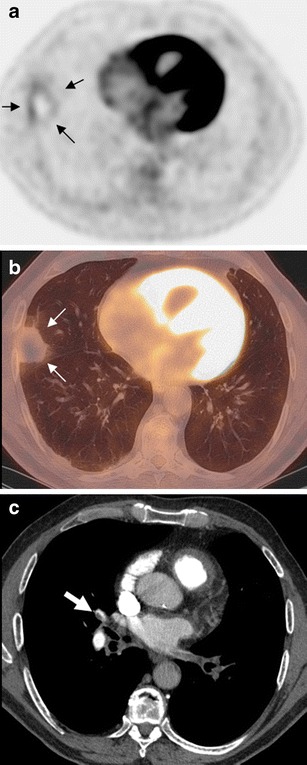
A 57-year-old man referred for workup of a solitary pulmonary mass (patient 5). FDG-PET/CT images showed a right subpleural consolidation with the rim sign (a, axial PET; b, axial fusion, *arrow*). MDCT confirmed acute pulmonary embolism (c, *arrow*) showing an adjacent subsegmental pulmonary embolism. Note the absence of any suggestive signs of pulmonary infarction on MDCT. Patient follow-up confirmed the absence of associated lung tumor
